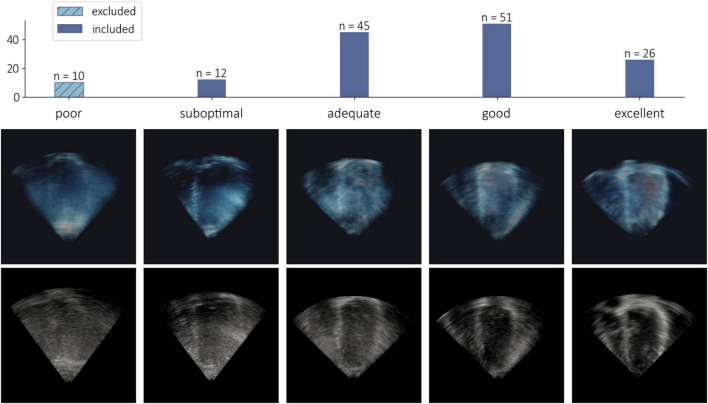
Examples of reconstructed 3D echocardiographic image volumes (visualized with an opacity transfer function on a blue-to-red colormap) and corresponding 2D mid-volume longitudinal slices (grayscale), showing variable quality (subjectively scored from poor to excellent). A total of 10 subjects were excluded from the study due to poor image quality.
